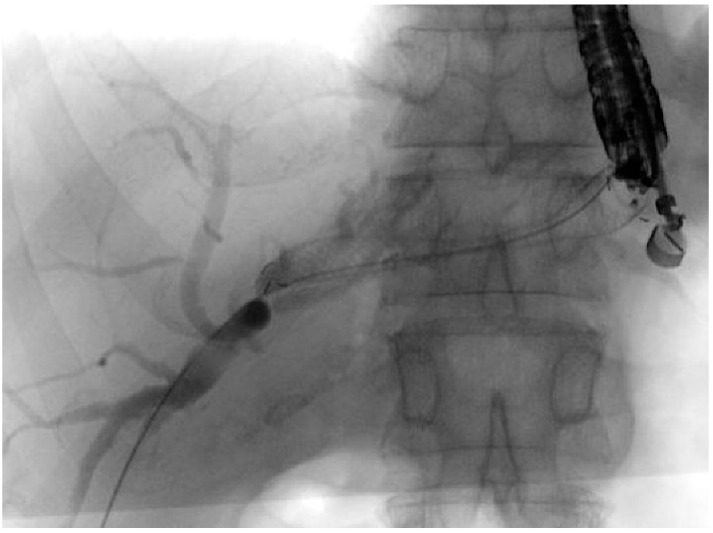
Endoscopic Ultrasound-Guided-Hepaticogastrostomy (EUS-HGS) with partially covered metal stent (PC-SEMS). The copyright of the image belongs to the authors.
